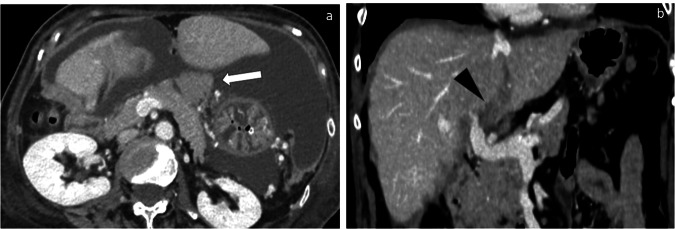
Axial (a) and coronal (b) CT images show infiltration of the lesser sac (white arrow) and of the hepatic hilum (black arrowhead)
